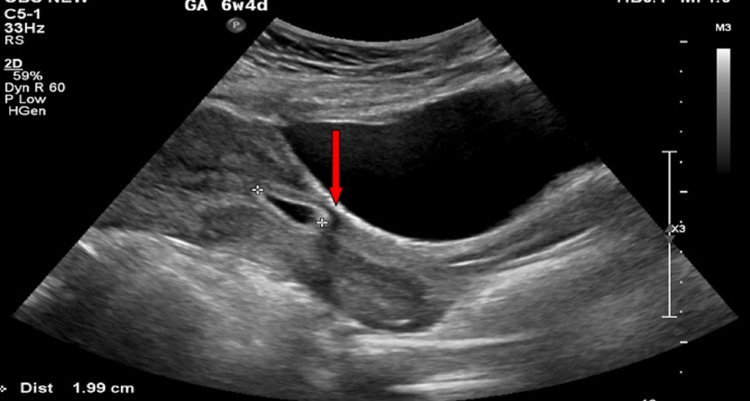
Transabdominal ultrasound showing 6 mm single small gestational sac with yolk sac of five weeks located in anterior part of lower uterine segment at cesarean scar, myometrium between bladder wall and gestational sac absent, and empty uterine cavity shown by red arrow.
